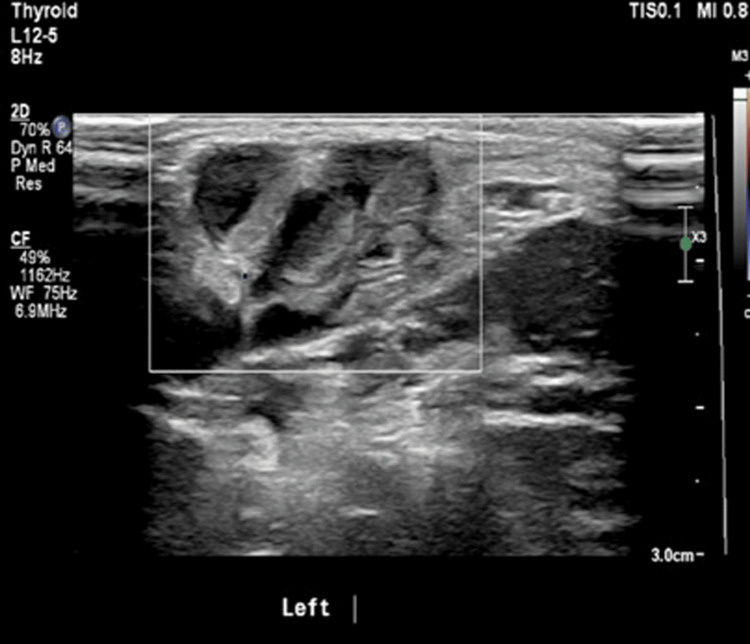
Color Doppler image of the swelling on the left side of the neck A color Doppler image obtained at the same level shows no obvious intralesional blood flow, suggesting a nonvascular origin of this lesion. Also note the lack of peripheral vascularity, which excludes the diagnosis of necrotic cervical lymph node/abscess.
